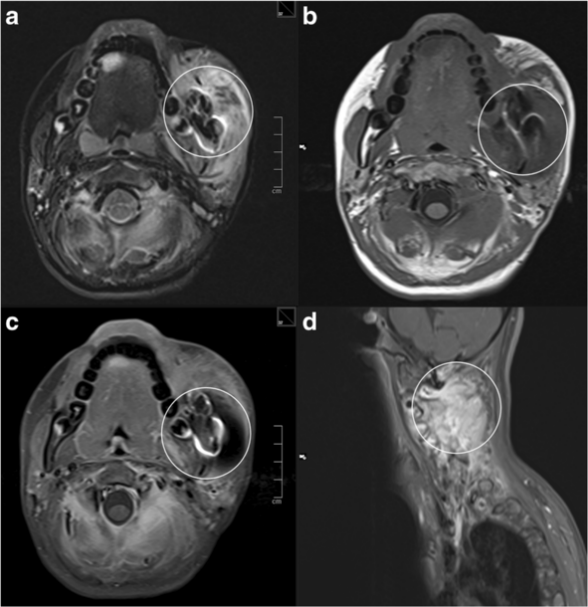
MRI-T2: Soft-tissue tumour with infiltration of the surrounding bone located at the left corpus and ramus of the lower jar (a, b, c - *circles*). Similar osseous and soft tissue lesions were detected in the cervical vertebrae 2–5 (d) (*circle*) including the vertebral arches and the transverse processes
